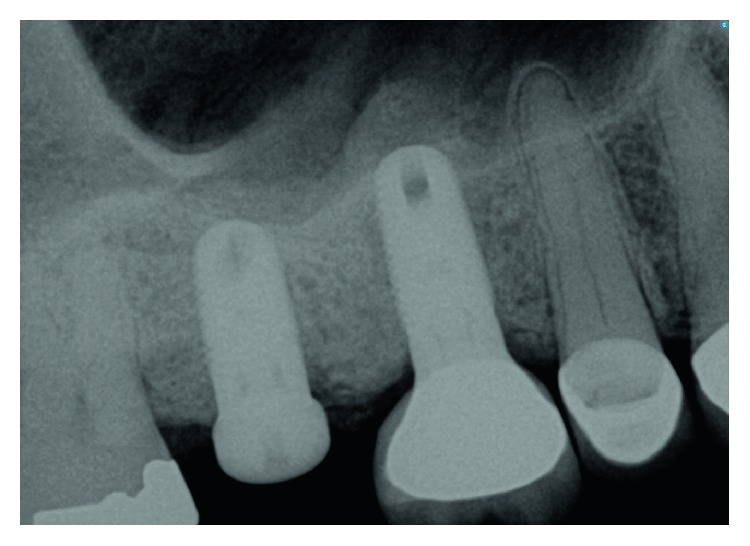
The same protocol of extraction, ridge preservation and subsequent implant placement, was followed successfully for site #2. The implant at site #3 did not show any signs of infection or inflammation, radiographically nor clinically.
